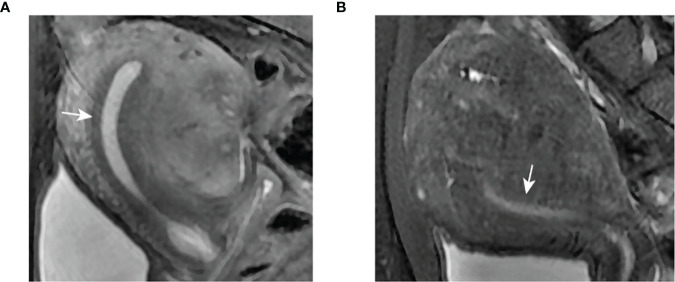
Clear demarcation of JZ appearance on MRI. (A) Thickened JZ in a patient with focal adenomyosis. (B) Thickened JZ in a patient with diffuse adenomyosis.
